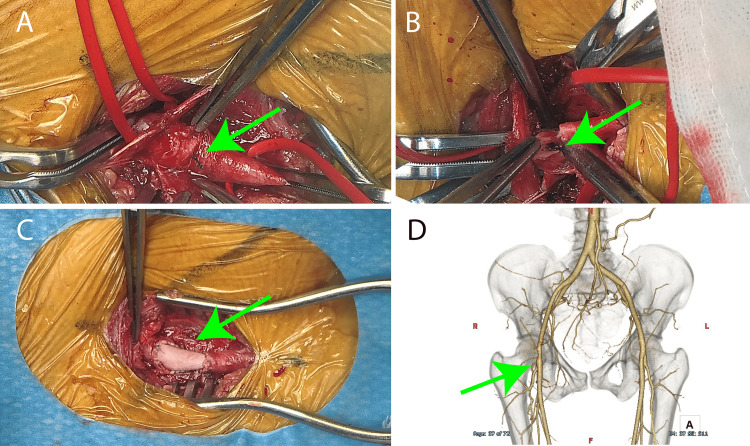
Intraoperative images and postoperative computed tomography (A) Intraoperative image. Pro-Glide knot was shown on the surface of the right femoral artery (green arrow). (B) Pro-Glide knot was sawn at the inner bottom of the right femoral artery (green arrow). (C) Right femoral artery plasty was conducted by patch (green arrow). (D) Postoperative image. Volume rendering computed tomography revealed the right femoral patch plasty (green arrow).
